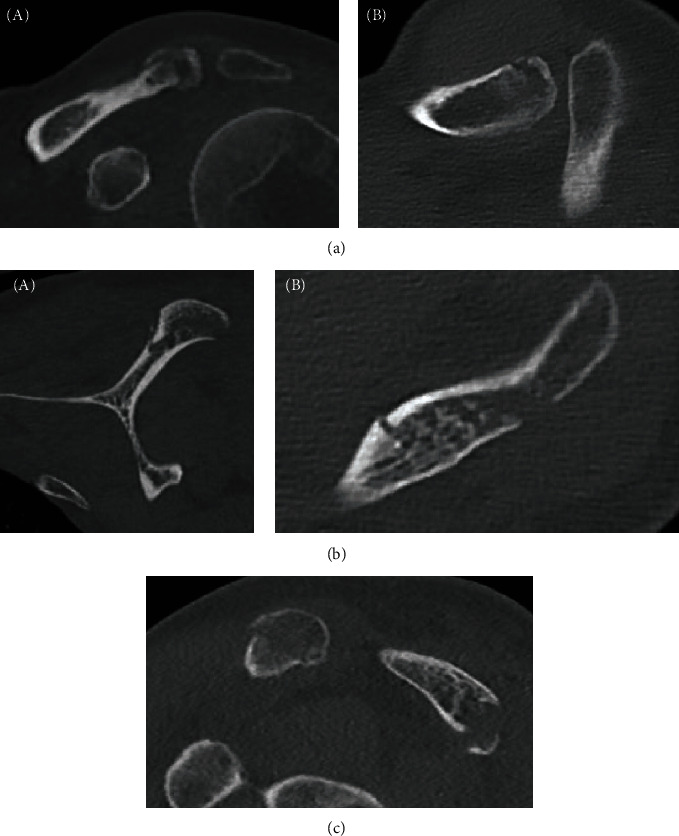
(a) Coronal (A) and transversal (B) view of the CT scan showing the distal clavicle fracture. (b) Coronal (A) and transversal (B) view of the CT scan showing the acromion fracture. (c) Sagittal view of the CT scan showing the acromion fracture and concomitant distal clavicle fracture.
